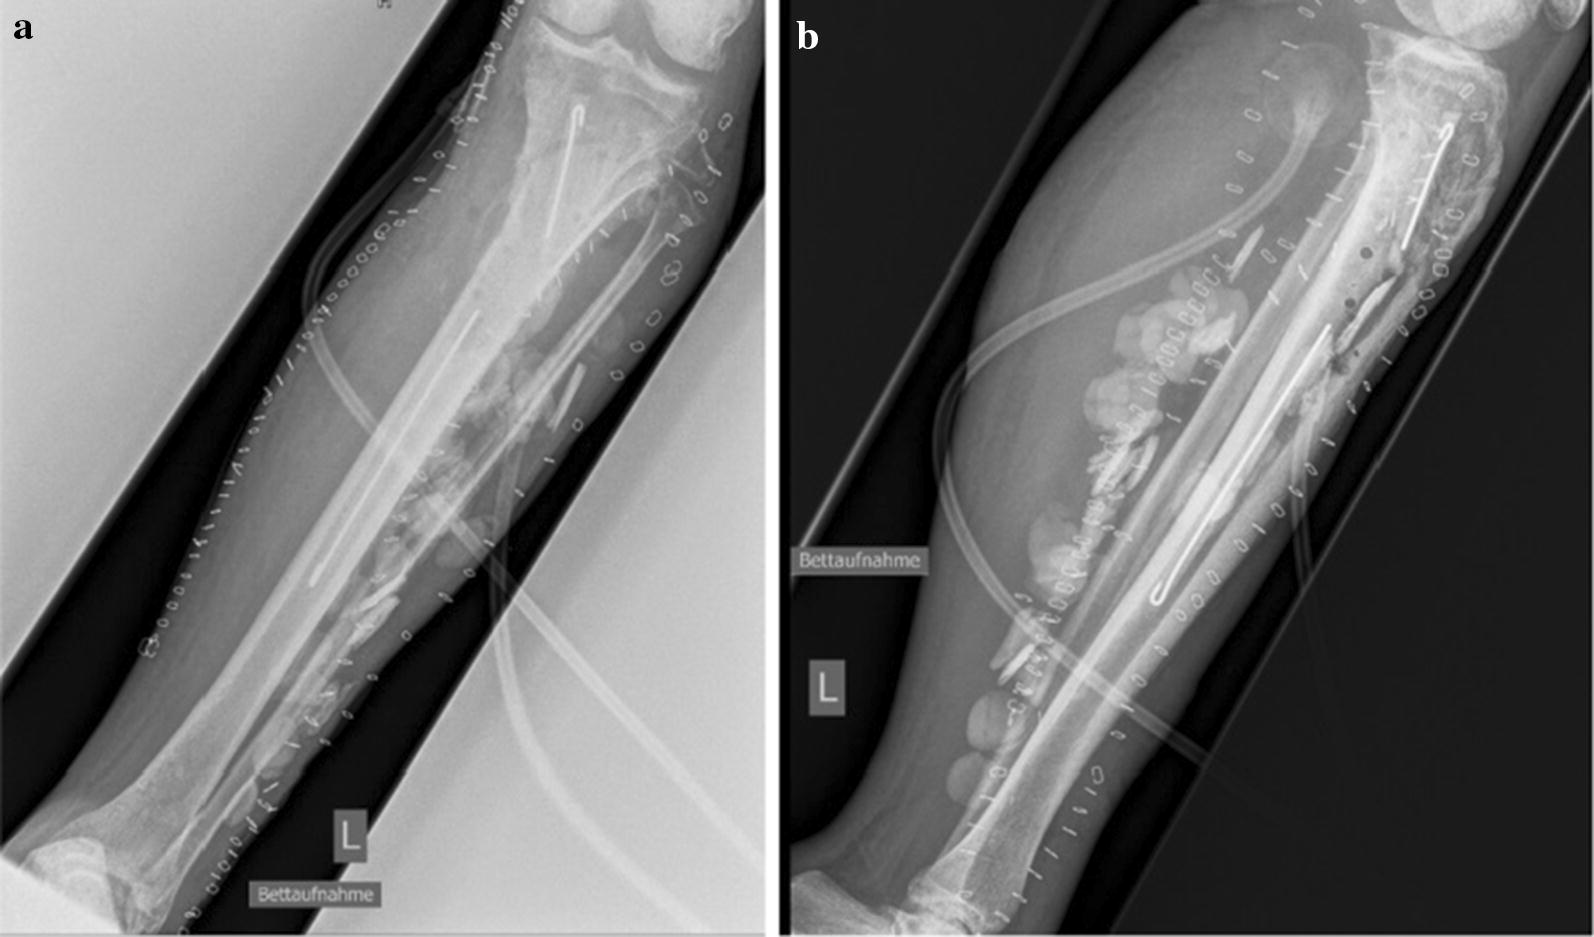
Plain X-rays showing reamed intramedullary zone, implanted spacer (sticks and chains) as well as negative pressure wound therapy in situ. a X-ray a.p. view and b X-ray lateral view
